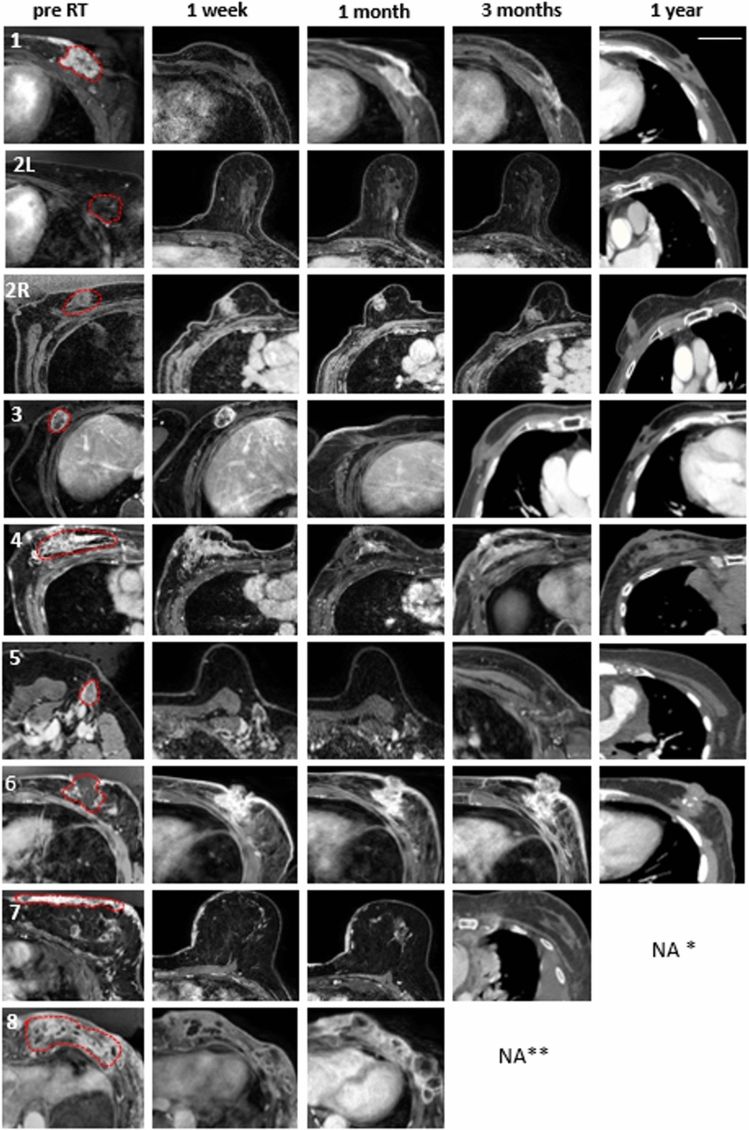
Individual tumour imaging before and after treatment The panel on the left represents pre-radiation target volume indicated, while the subsequent images show radiological outcomes with T1-weighted contrast-enhanced MRI at 1 week, 1 month, 3 months, and CT image at 1 year after treatment. *Patient 7 died of metastatic disease at 9 months (1-year follow-up not available), Patent 8 died of metastatic disease at 3 months (3 months and 1-year follow-up not available). The scale on the top right figure represents an approximate size of 5 cm.
